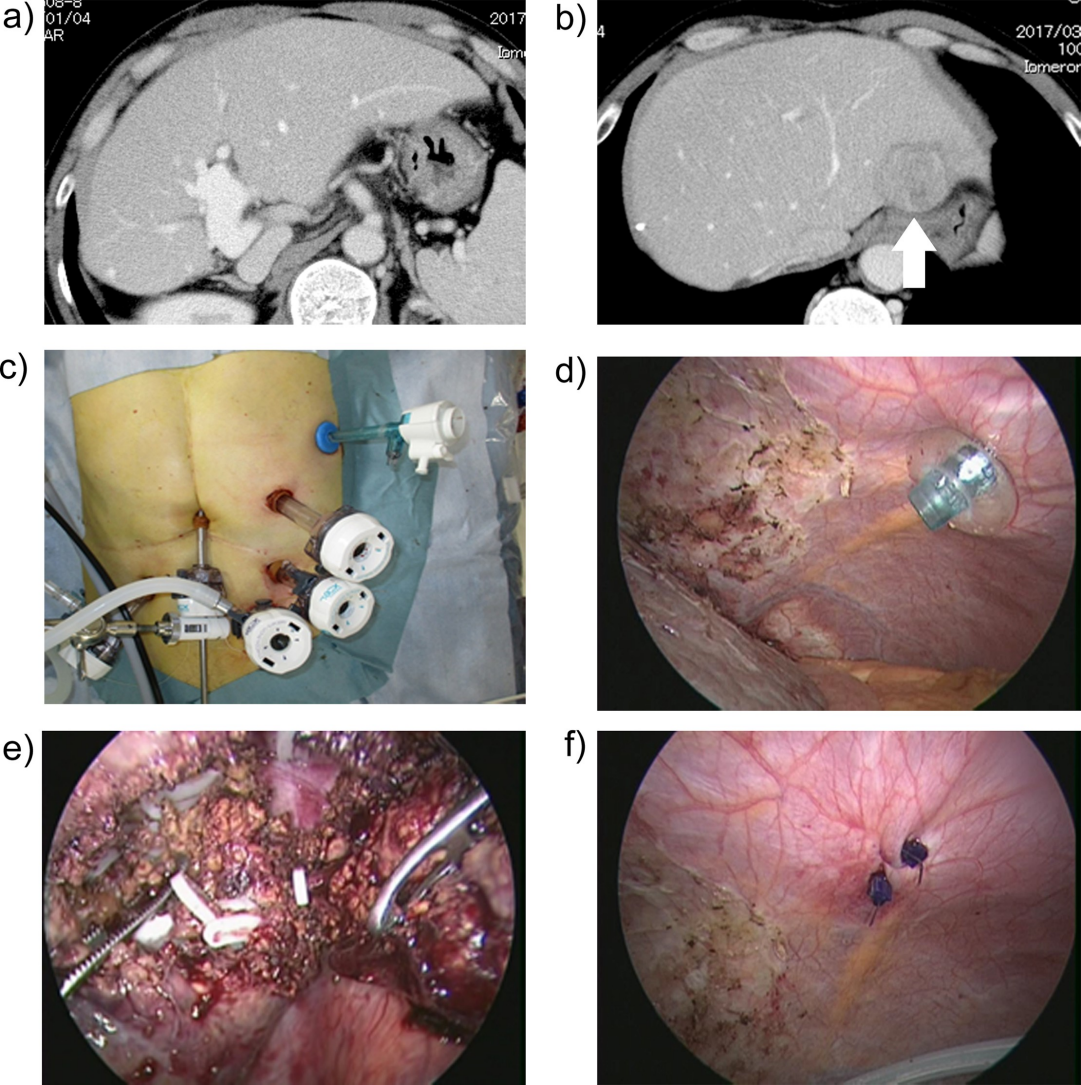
Application of a left intercostal diaphragmatic port in repeated hepatectomy. a), b) Enhanced computed tomography shows a recurrent hepatocellular carcinoma located in segment II after open right hemi-hepatectomy. An arrow indicates the recurrent tumor. c) Extracorporeal view of the left intercostal port.d) Intracorporeal view of the left intercostal port.e) View during repeated hepatectomy. f) Suture of the port hole.
